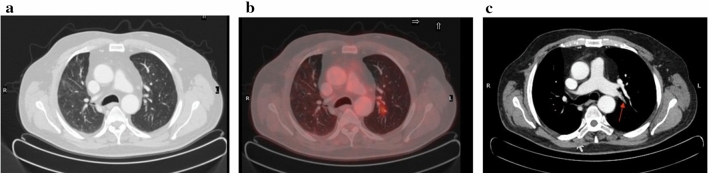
18F-FDG PET/CT performed in a 64-year-old patient two months after the acute phase of COVID-19: transaxial images of contrast-enhanced CT of the thorax with pulmonary (a) or mediastinal (c) window and corresponding PET/CT fusion images (b). Focal FDG uptake was observed in the left paramediastinal region (b) corresponding to an endoluminal ipodensity in the superior branch of the left pulmonary artery (c, arrow)
